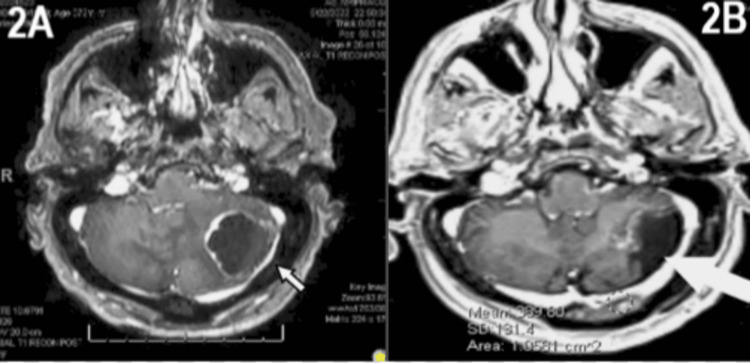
Brain Imaging Showing Cerebellar Metastases at Different Stages of Treatment 2A: Original MRI of the brain taken immediately following diagnosis, which revealed cerebellar metastasis. This image was taken prior to initiating treatment to monitor for possible brain metastases, and, upon review, it was concluded that the malignancy had metastasized to the cerebellum at the time of presentation. 2B: Follow-up MRI was done after the patient received treatment with both SRS (stereotactic radiation surgery) for the brain metastases and eight months of osimertinib to treat the patient’s lung tumor and metastases. This imaging showed growth and further depth of invasion of the original cerebellar mass observed eight months prior to the time of diagnosis.
